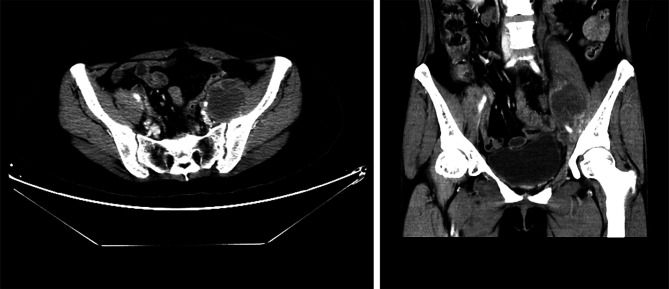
Pelvic MRI on April 1, 2019. Coronal view showing an anterior abdominal wall metastasis after niraparib administration for 20 months.
